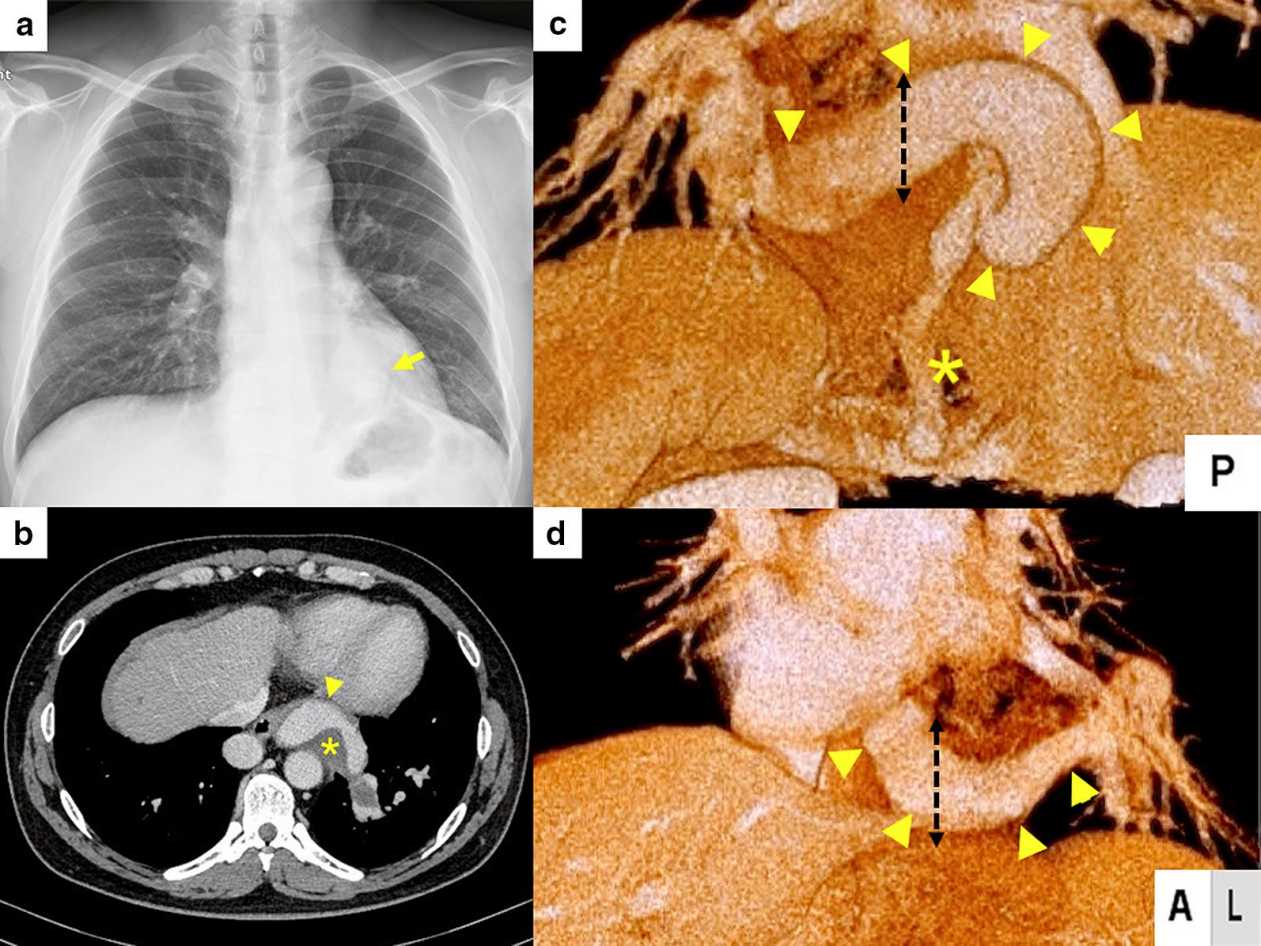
Preoperative chest X-ray and computed tomography images. a Chest X-ray image showing abnormal consolidation in the left lower lung field (yellow arrow). b Enhanced computed tomography revealed the presence of an aberrant artery with a maximum diameter of 33 mm (yellow arrowheads) with a thrombosed lumen (asterisk). c Three-dimensional computed tomography from posterior view revealed an aneurysmal aberrant artery (yellow arrowheads) arising from the celiac artery. We resected the aberrant artery with pulmonary ligament (black dotted line). After surgery, we performed coil embolization on the residual aberrant artery (asterisk). d Three-dimensional computed tomography from anterior view showed that the aneurysmal aberrant artery (yellow arrowheads) arose from the celiac artery and was flowing into the left lower lobe along the pulmonary ligament forming an aneurysm. It was divided along with the pulmonary ligament (black dotted line)
